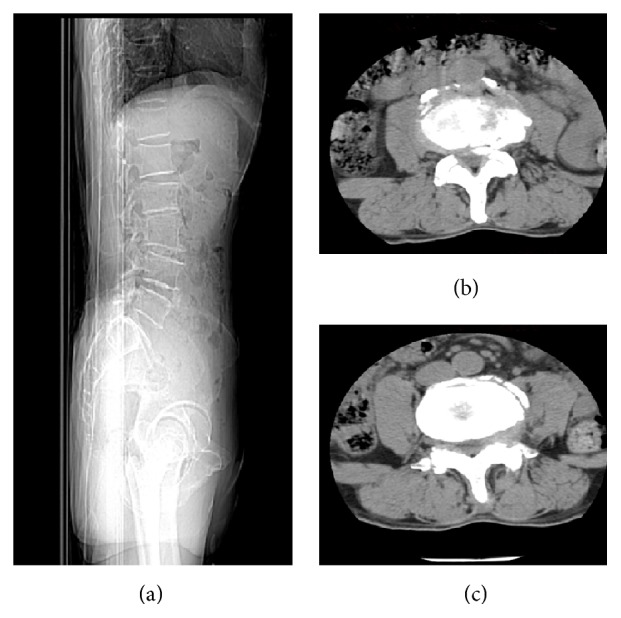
The sagittal (a) and coronal CT images of L3-4 (b) and L4-5 (c) revealed lumbar stenosis combined with intervertebral disc herniation at both levels and combined lumbar facet joint degenerative changes.
